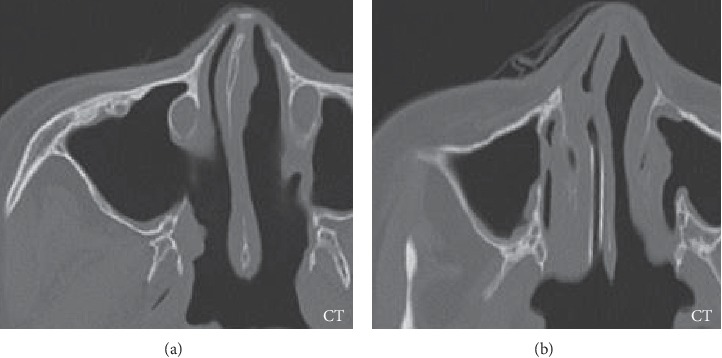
Post-ESS images. CT: Resected tumor in the right maxillary sinus.
